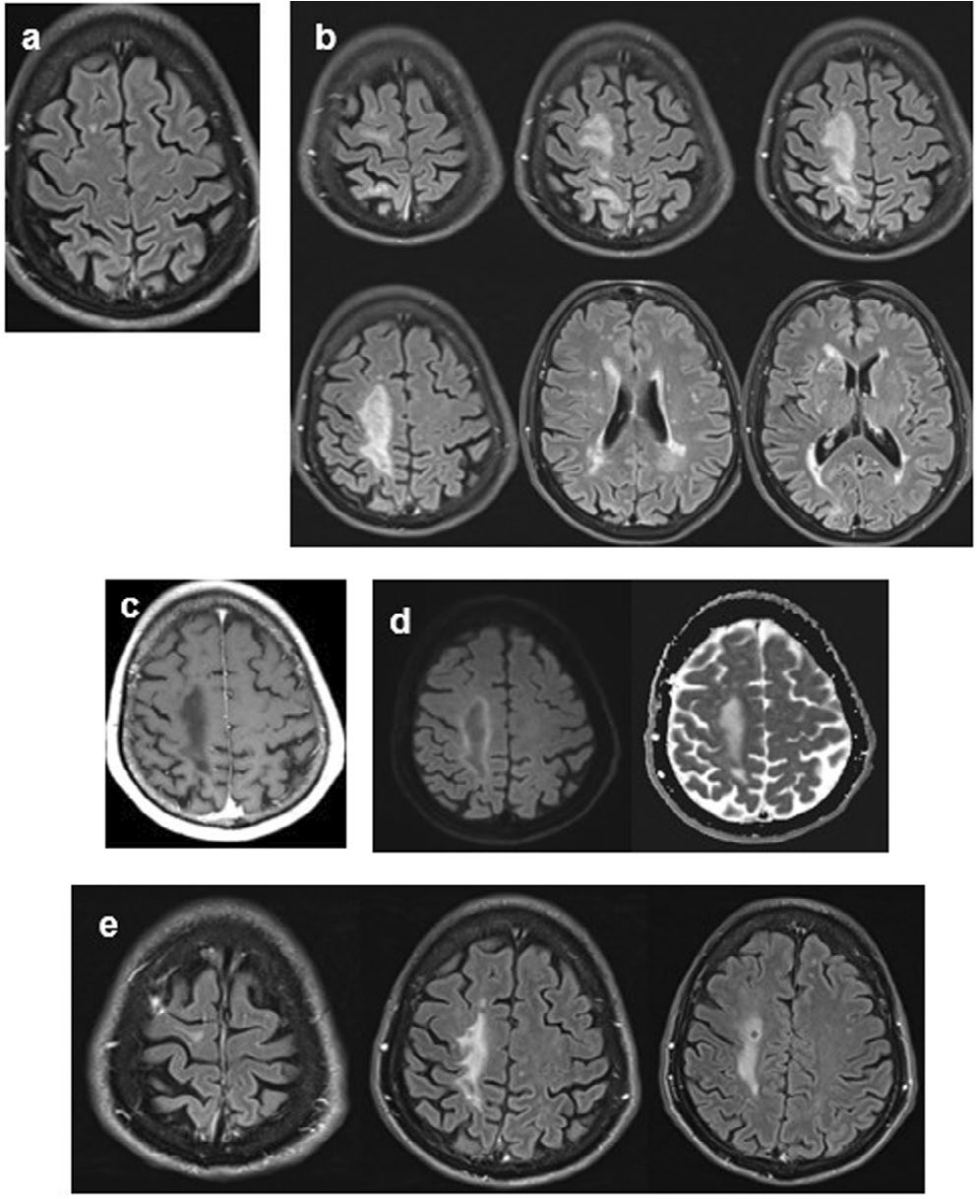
FIGURE 1 Case 1. Baseline brain MRI (a). Right fronto‐parietal juxta‐subcortical white matter lesion hyperintense on FLAIR (b), with no contrast enhancement (c) and mild peripheral diffusion restriction on DWI (d). Reduction in size in follow‐up MRI (d).
